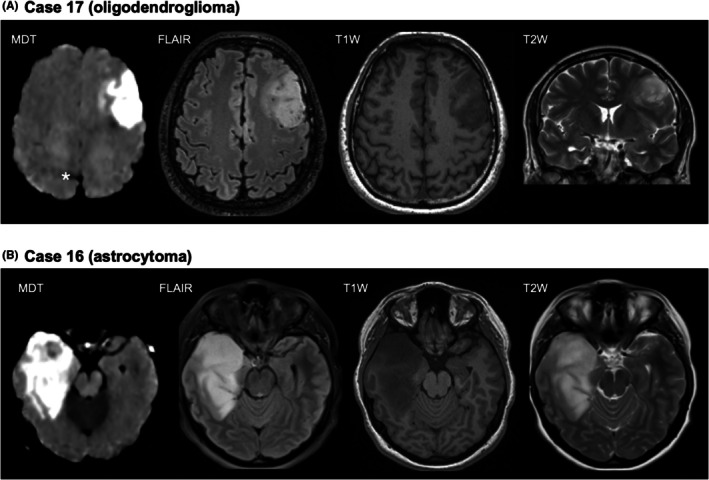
Oligodendroglioma and astrocytoma. Panel A shows case 17 with a grade II oligodendroglioma. MDT and FLAIR both demonstrated strong hyperintensity, with sharper delineation on MDT. T1W showed hypointensity and T2W hyperintensity. Panel B shows case 16 with a grade II–III astrocytoma. MDT demonstrated a strong hyperintensity closely following the FLAIR signal, again with sharper delineation compared to FLAIR. An asterisk marks a fat artifact. Again, T1W showed hypointensity and T2W hyperintensity in the same region.
